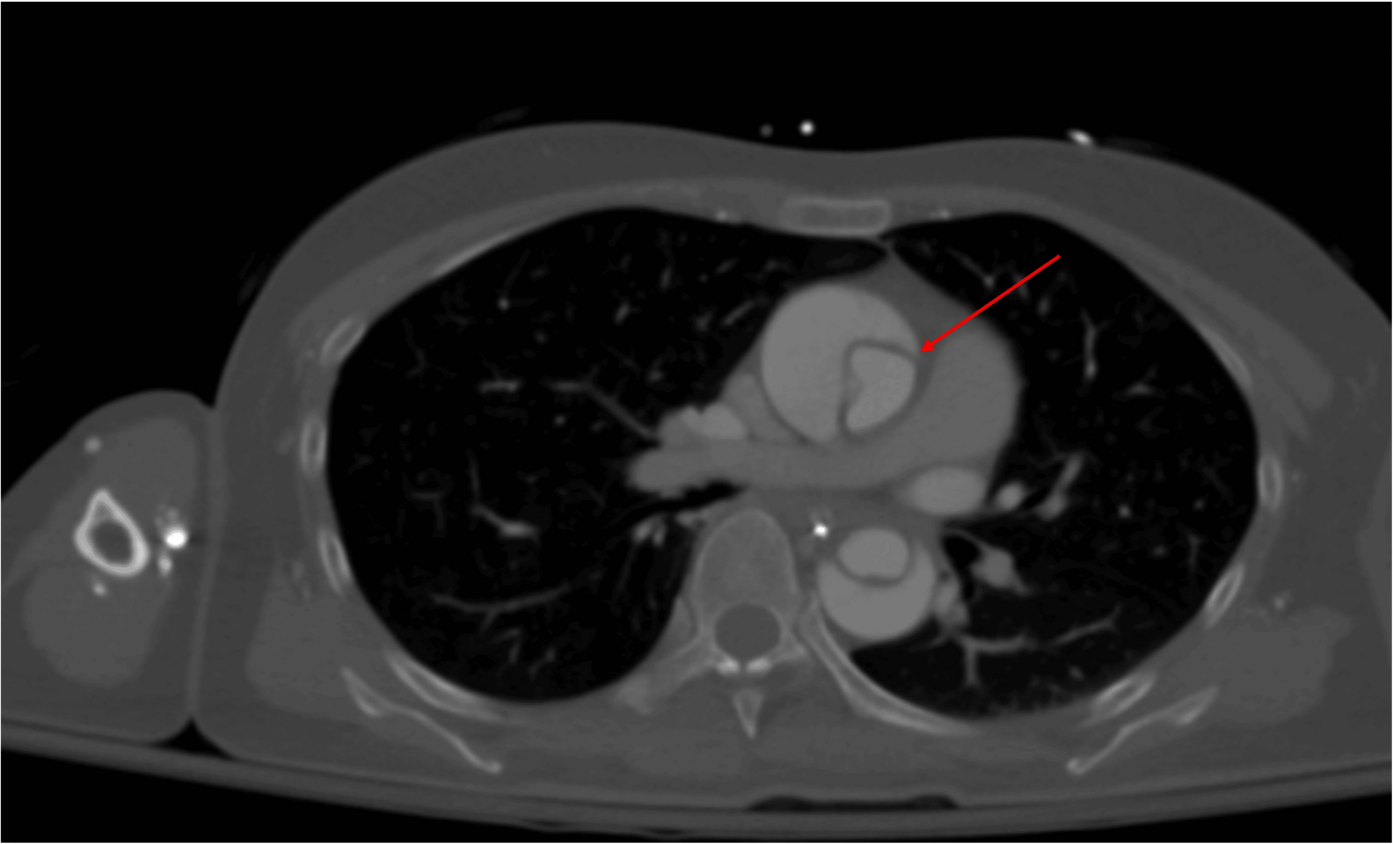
Axial CT angiogram of the chest demonstrating a Stanford Type A aortic dissection with visible intimal flap and true and false lumens in the ascending aorta.
